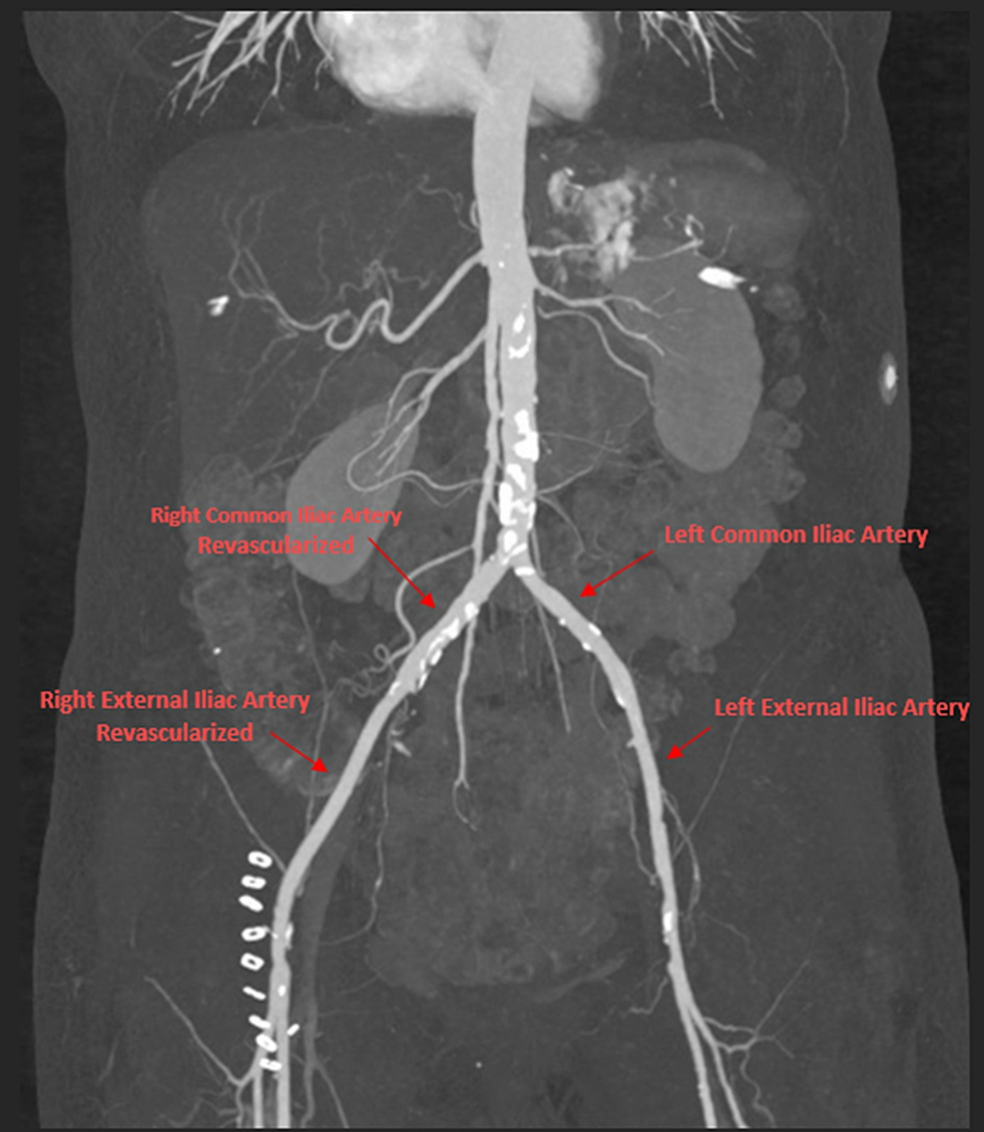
CTA of the abdominal aorta with runoff after thrombectomy showing revascularization of the right common and right external iliac arteries. CTA: computed tomography angiography
